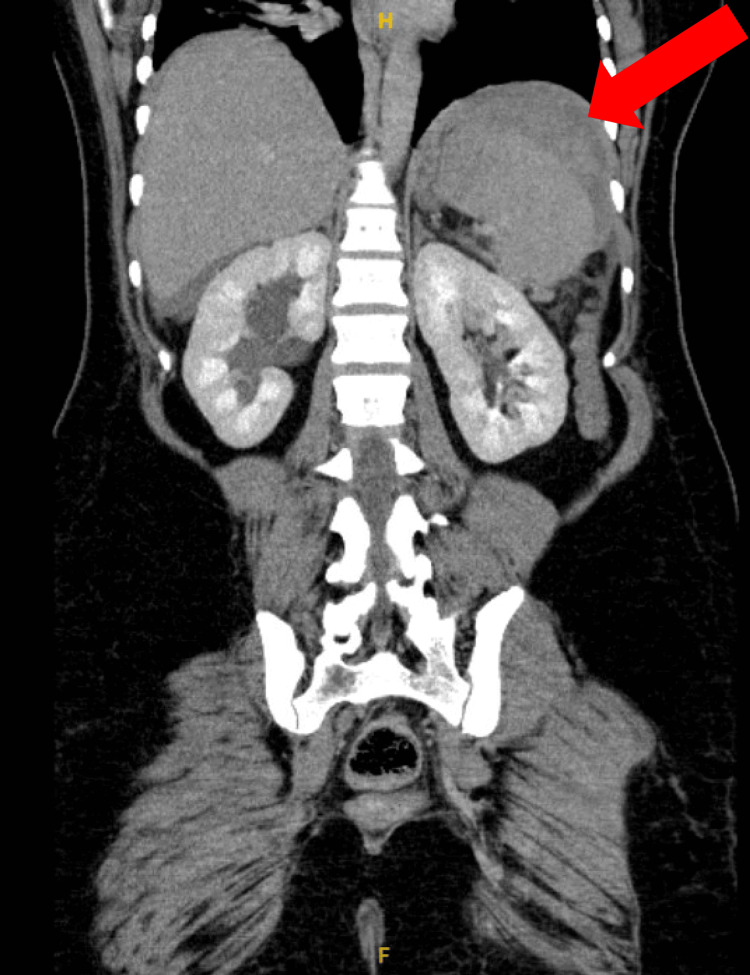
Contrast-enhanced CT of the abdomen and pelvis demonstrating a large perisplenic hematoma (coronal view) The red arrow indicates irregularity of the inferior aspect of the spleen and a large amount of perisplenic hypodense fluid, consistent with hemoperitoneum.
